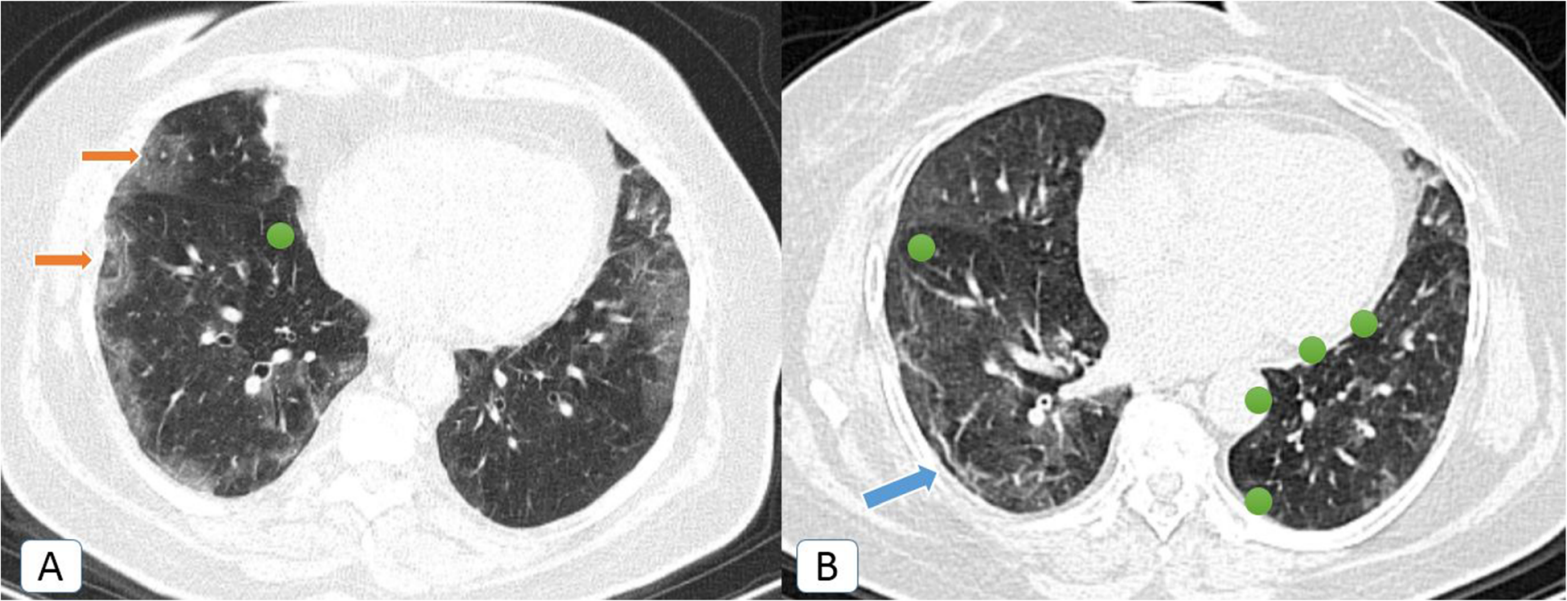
COVID-19 infection atypically presented by head-cheese pattern (ground-glass attenuation alternating with air trapping): a 48-year-old female COVID-19 patient. A Initial axial chest CT (lung window) revealed multiple sub-pleural ground glass patches (orange arrows) of COVID-19 infection with a nearby medial basal area of air trapping (green circle) … “Head-cheese pattern.” B Follow up axial chest CT after 3 weeks (lung window) revealed healing of the ground-glass patches by curvilinear fibrotic bands (blue arrow) with persistent areas of air trapping
